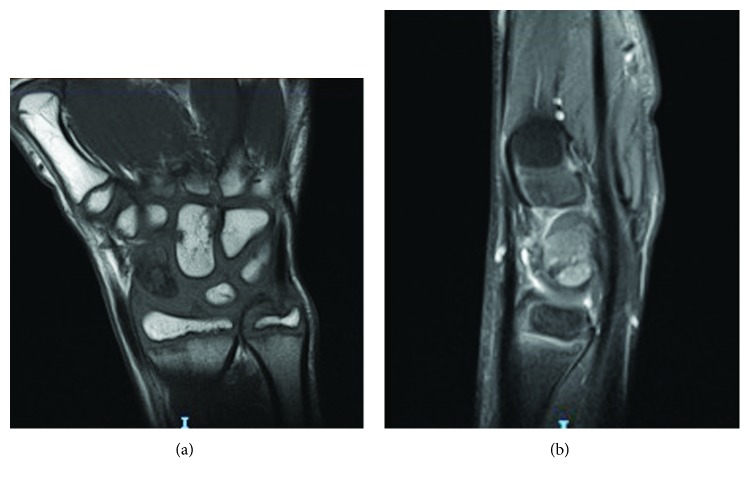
(a) Coronal T1-weighted magnetic resonance (MR) image of the right wrist showing diffuse loss of signal throughout the scaphoid bone that is concerning for osteonecrosis; (b) sagittal fat-suppressed PD-w MR image of the right wrist. The presence of a fracture line is indicative of nonunion.
